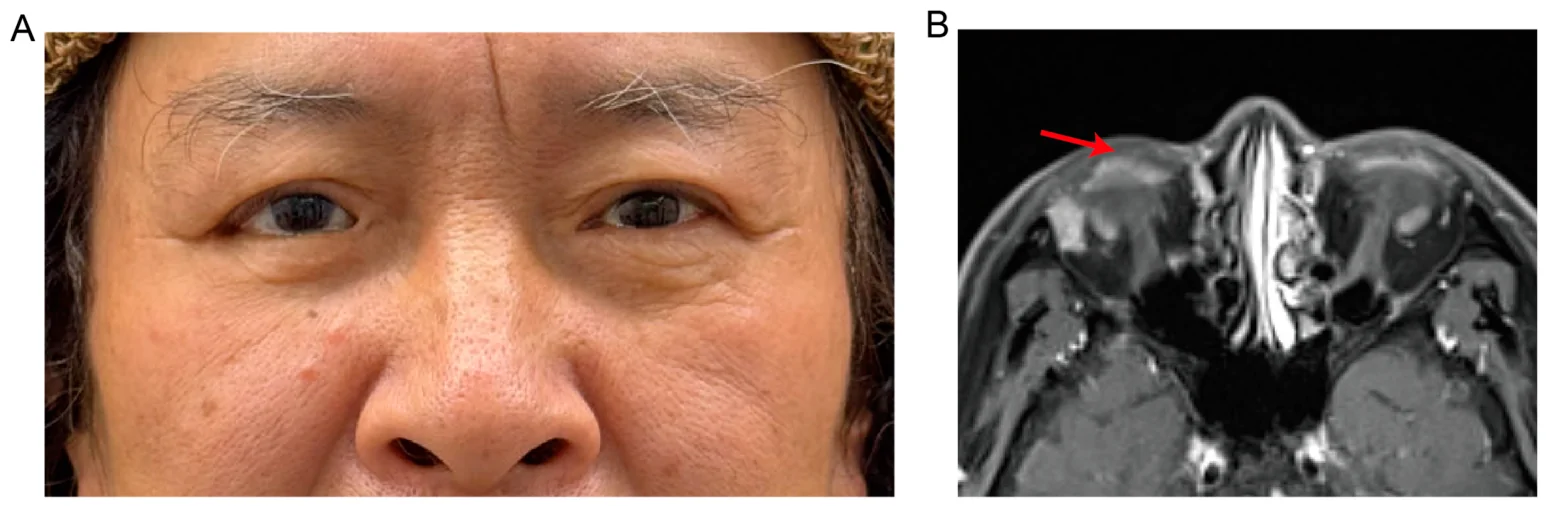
Long-term outcome. (A) Four years after stage 1 and 3.5 years after stage 2. (B) Enlarged follow-up MRI view of the prior tumor bed/reconstructed lower-eyelid region; the red arrow indicates the retained cartilage graft, and no recurrence was identified.
